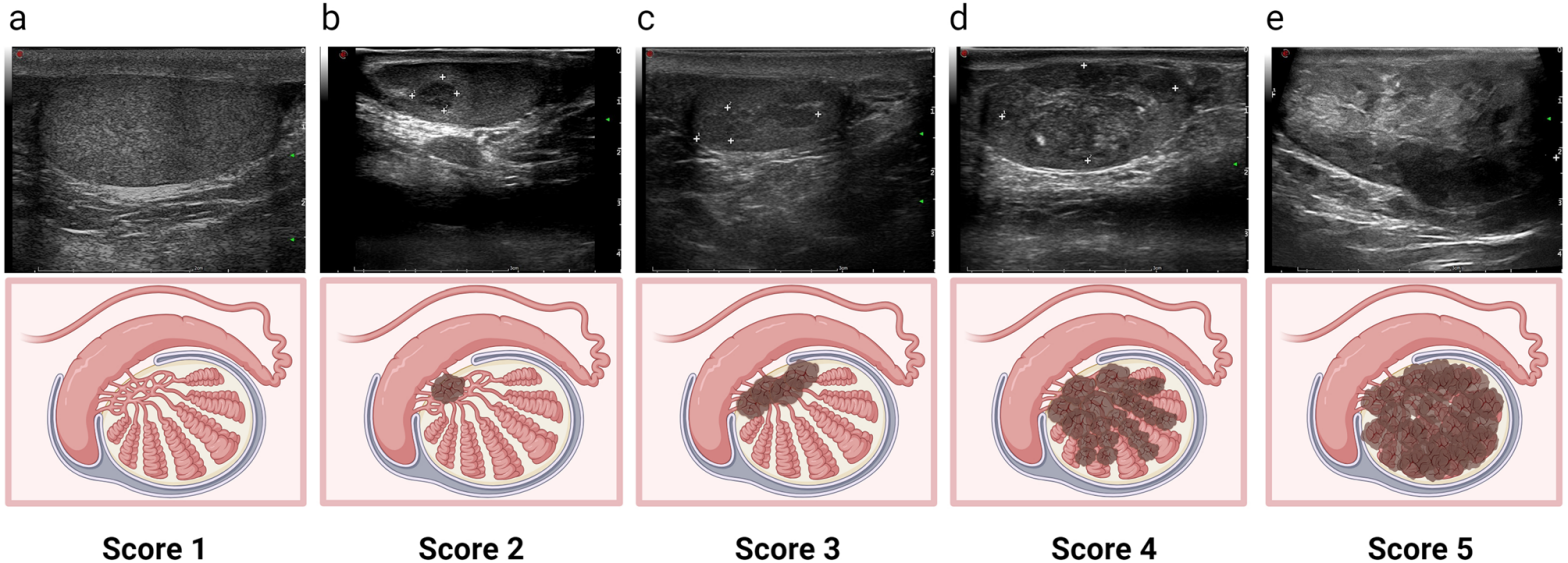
Typical images of testicular adrenal rest tumor (TART) involvement range scores. (a–e) show testicular B-mode ultrasound images of five male patients with congenital adrenal hyperplasia (CAH), aged 28, 41, 13, 11 and 17, respectively. (a) Score 1: Not detectable by scrotal ultrasound; (b) Score 2: Aggregated oval lesions in the mediastinum, involving no more than 1/3 of the sagittal section of the testis; (c) Score 3: The lesion fills the mediastinum and does not involve more than 1/3 of the transverse and sagittal of the testis; (d) Score 4: Under the circumstance of all the mediastinal involvement, the lesion on the transverse involves more than 1/3 of the testis with residence of normal testicular tissue; (e) Score 5: Diffusely growing lesion, no normal testicular tissue visible.
